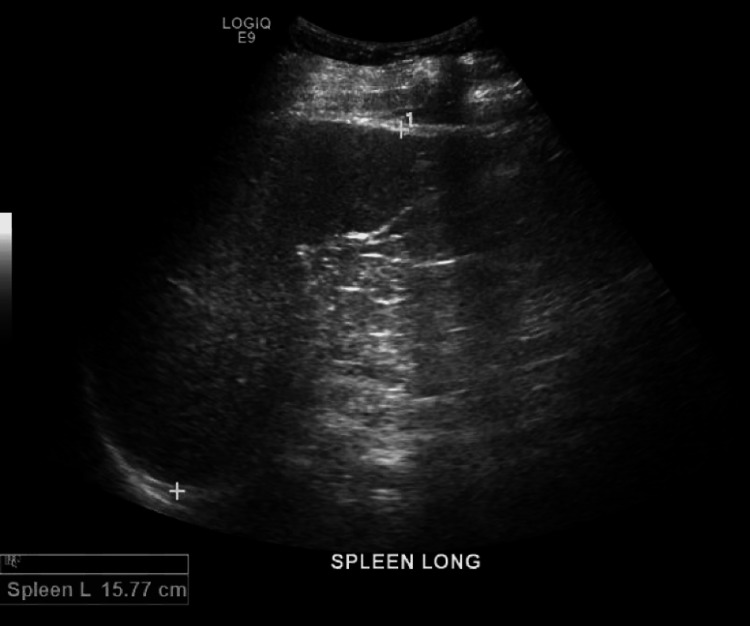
Splenomegaly found on patient’s abdominal ultrasound
